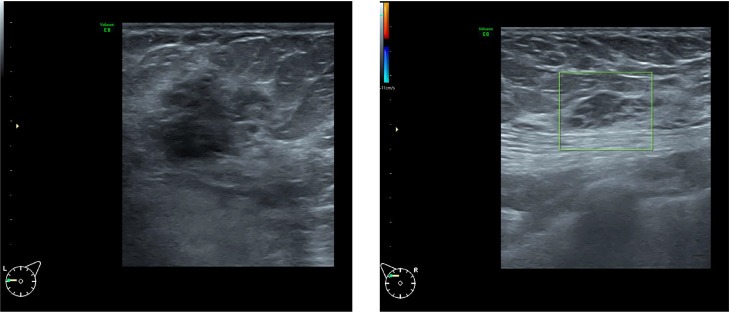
Ultrasound examination demonstrated a heterogeneous, hypoechoic, irregular, microlobulated, antiparallel mass in the left breast ultrasound examination also demonstrated a similar, smaller mass in the right breast.
